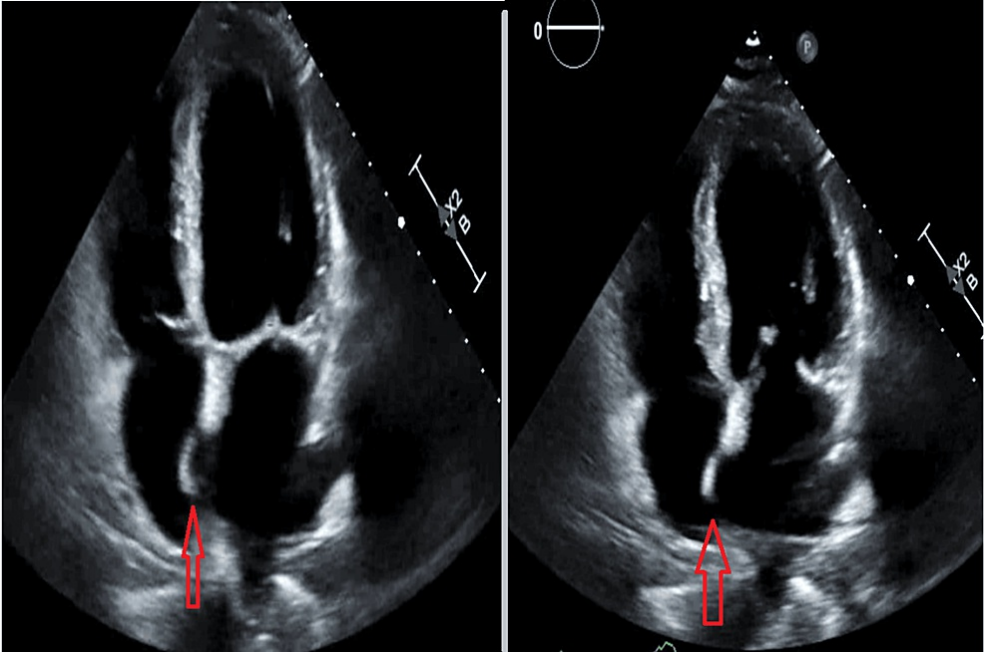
TTE (apical four-chamber view). Arrows showing excursion of the atrial septum into the right atrium. This is compatible with an atrial septal aneurysm. TTE, transthoracic echocardiography
